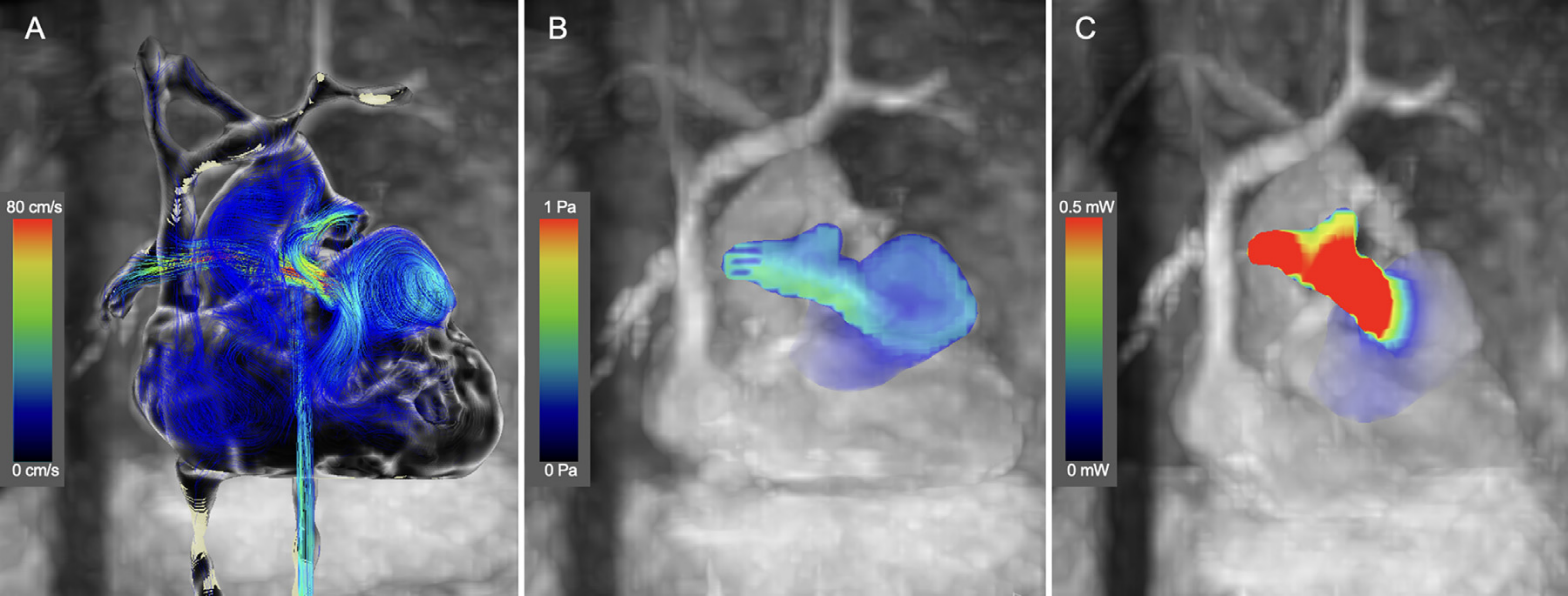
(A-C) Postinterventional 4D Flow MRI. (A) The streamline shows residual vortex flow at the aneurysm. (B) The peak WSS was 0.16 Pa, the average WSS was 0.09 Pa. (C) A peak EL of 0.33 mW and an average EL of 0.17 mW. The WSS and EL within the RVOT aneurysm was unchanged compared to before balloon dilation.
